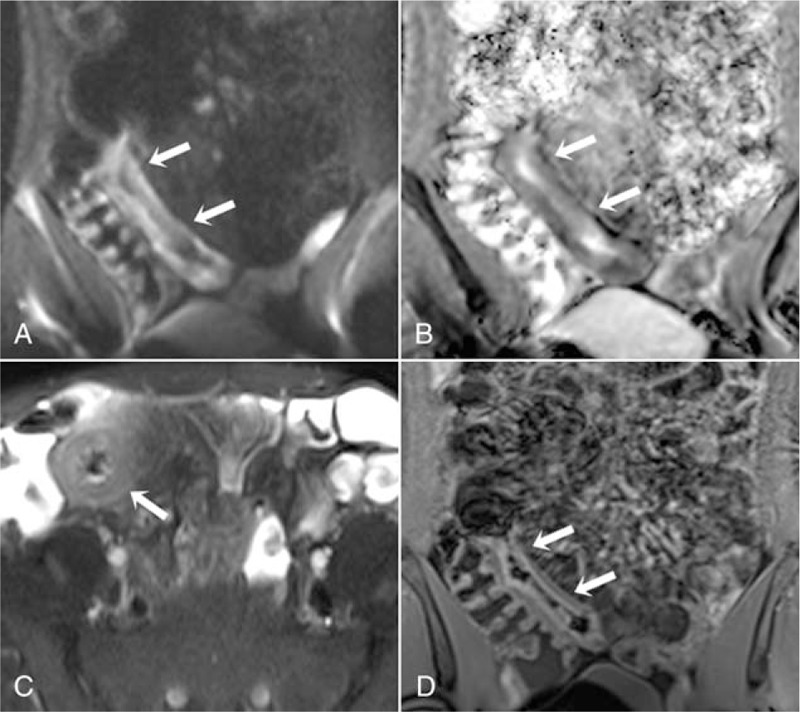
A 30-yr-old male patient with active Crohn disease. Diffusion restriction is observed in the distal and terminal ileum in the coronal DWI (A) and ADC map (B) (arrows) (scored 2 points on the MRAI). On the axial T2-weighted images (C) of the same patient, wall thickness, signal increase secondary to oedema, and perimural fluid ring are observed in the terminal ileum (arrow). On the coronal contrast-enhanced T1-weighted images (D), mucosal hyperenhancement is seen (scored 2 points on the MRAI). ADC = apparent diffusion coefficient, DWI = diffusion weighted imaging, MR = magnetic resonance.
